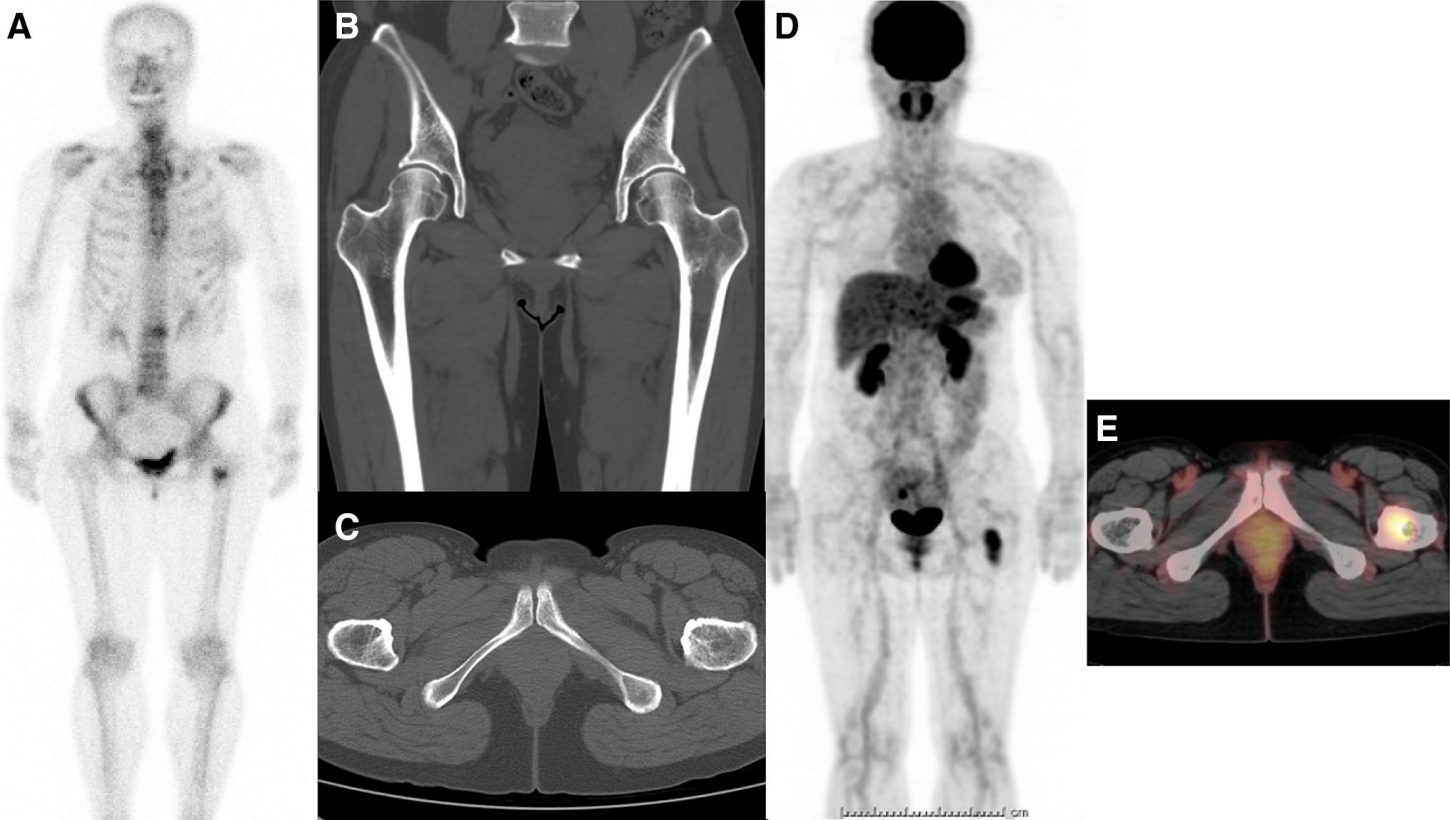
A 51-year-old female had right breast cancer surgery 10 years ago. She showed a tumor marker elevation (carcinoembryonic antigen), and bone scintigraphy (BS) was performed. A hot spot was shown in her left femur (around lesser trochanter) on BS (a). However, following CT could not demonstrate the lesion (b coronal, c axial). Therefore, FDG-PET/CT study was carried out, and an intense FDG uptake was noticed on her left femur (d whole-body FDG image, e fusion axial image). CT-guided bone biopsy revealed the lesion as metastasis pathologically
